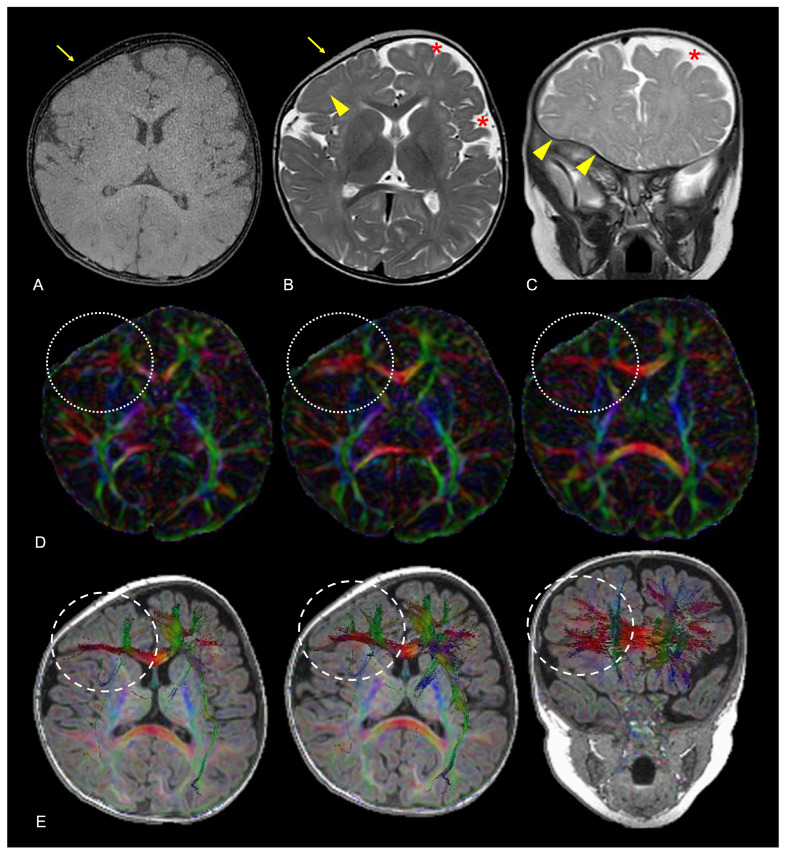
Axial black-bone MR image (A), axial TSE T2W image (B) and coronal TSE T2W image (C) of a 9-month-old female patient diagnosed with anterior plagiocephaly due to premature fusion of the right coronal suture (yellow arrows); aberrant adaptation of the right frontal lobe nervous tissue is indirectly demonstrated by the anomalous orientation of the frontal and frontal-insular sulci both on the axial and coronal planes (yellow arrowheads) compared to the left side, coupled to the asymmetric representation of adjacent CSF spaces (red asterisks). Axial FA maps (D) and color-coded representation of diffusion tensors on axial and coronal reconstructions superimposed on 3D-T1W images (E) confirmed altered fractional anisotropy (white dotted lines) and aberrant white matter fiber orientation (white dashed lines) due to white matter structural adaptive changes in the corresponding area. Color legend: red for left–right; blue for superior–inferior; and green for anterior–posterior.
